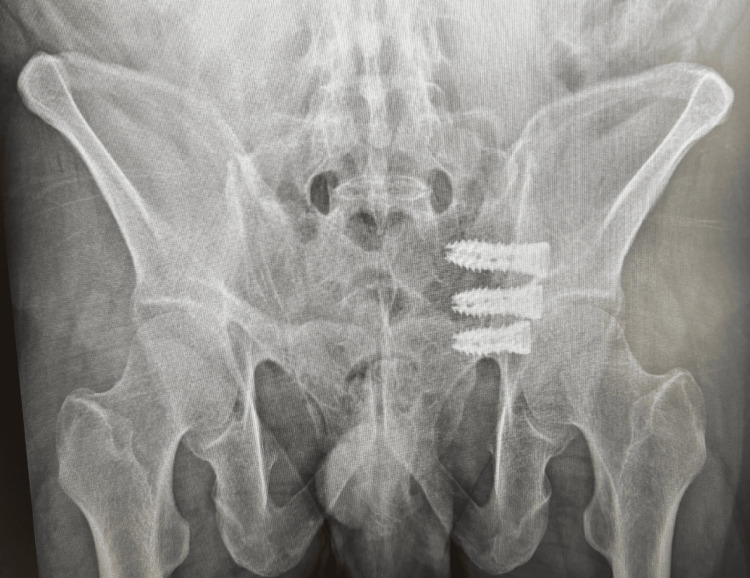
Postoperative Pelvic Outlet Radiograph Follow-up imaging at six months showing maintained implant position. The implants remain stable with no evidence of migration or lucency.
